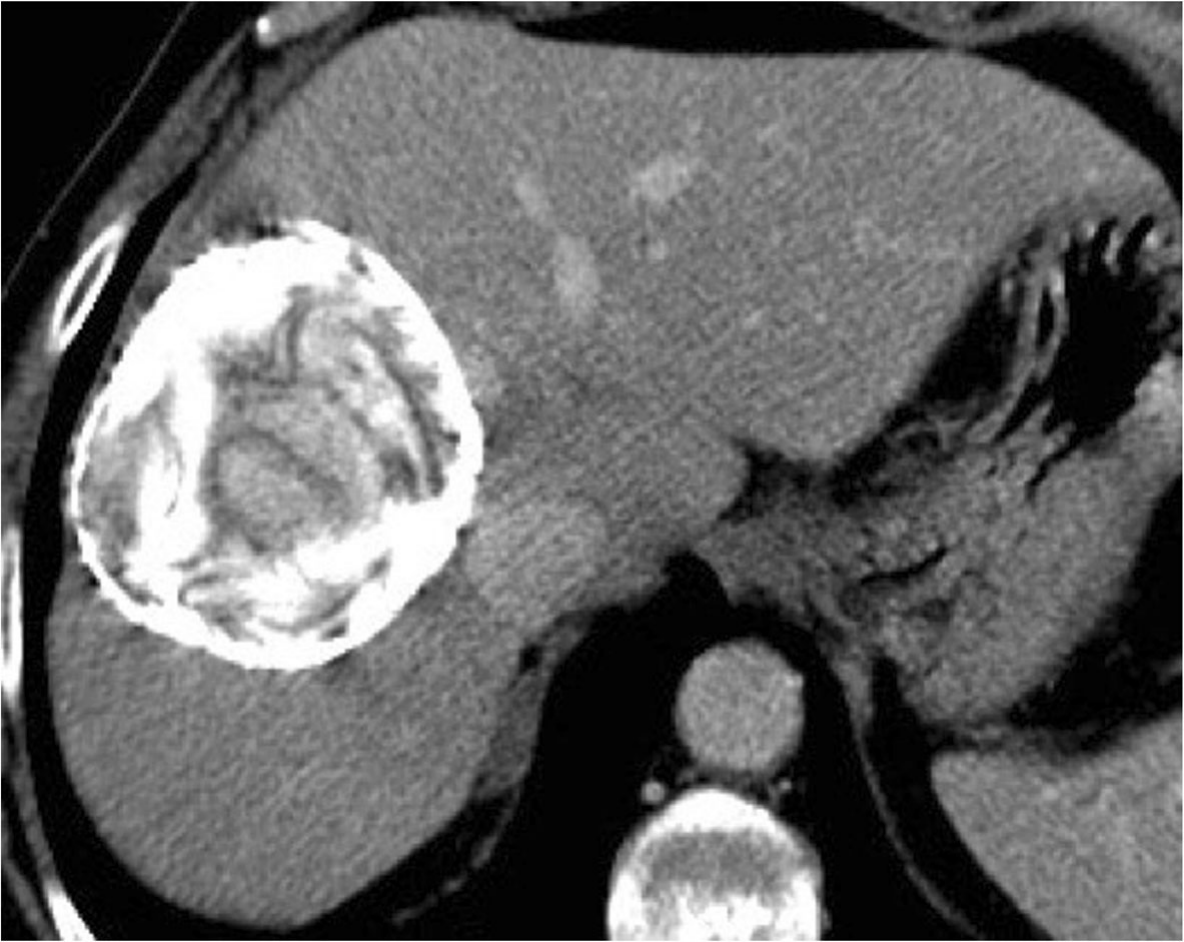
A 65-year-old woman with contained rupture hydatid cyst due to response to therapy. Contrast-enhanced CT on portal venous phase shows the serpentine linear membrane within an almost completely calcified cyst
